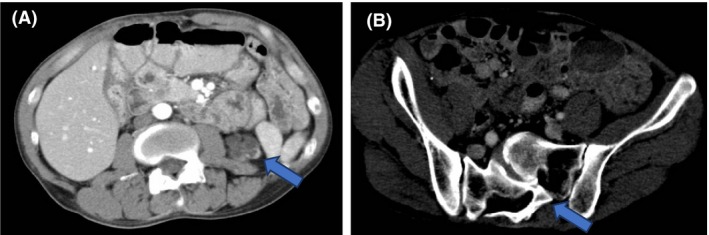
A CT image showing the characteristic of VATER association. (A) Renal malformations (hypoplasia of the left kidney: blue arrow, right kidney aplasia) and (B) vertebral body abnormality are recognized. These are two of the five signs that comprise VATER association.
